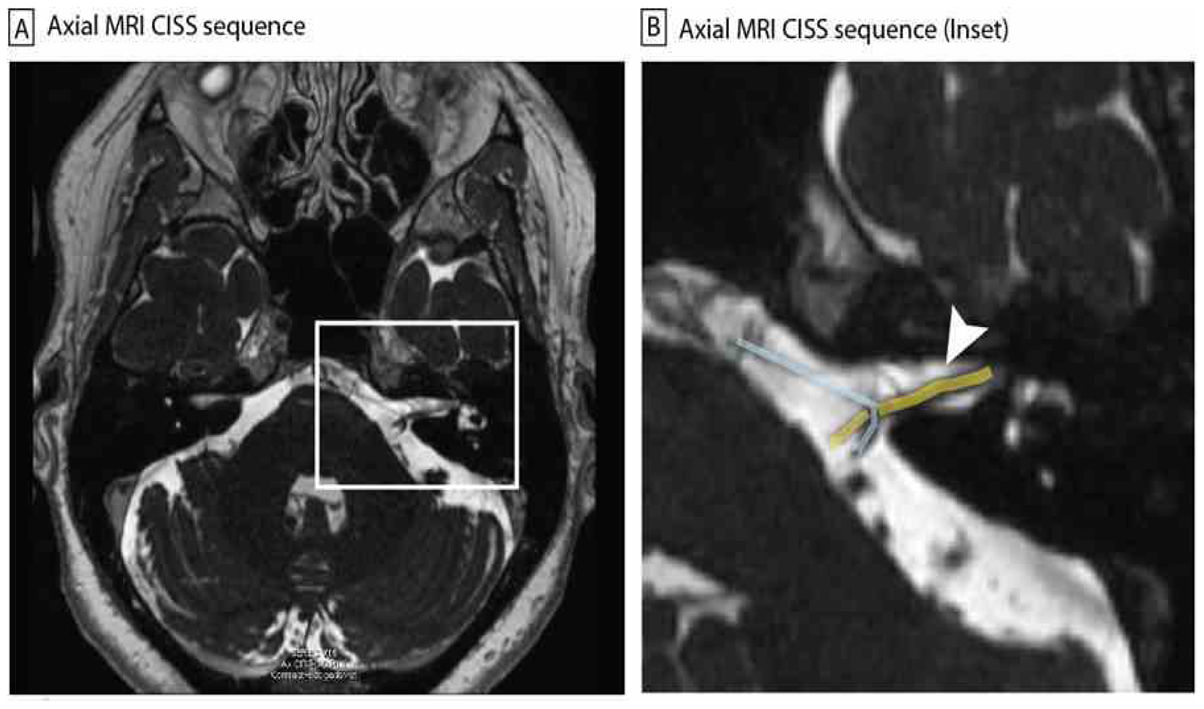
Axial constructive interference in steady state (CISS) sequence MRI imaging showing vascular compression at the internal auditory canal (IAC) (A). Inset (B) is magnified view of the left IAC (white arrow), with the nerves (yellow) and vessel (blue) highlighted for emphasis.
